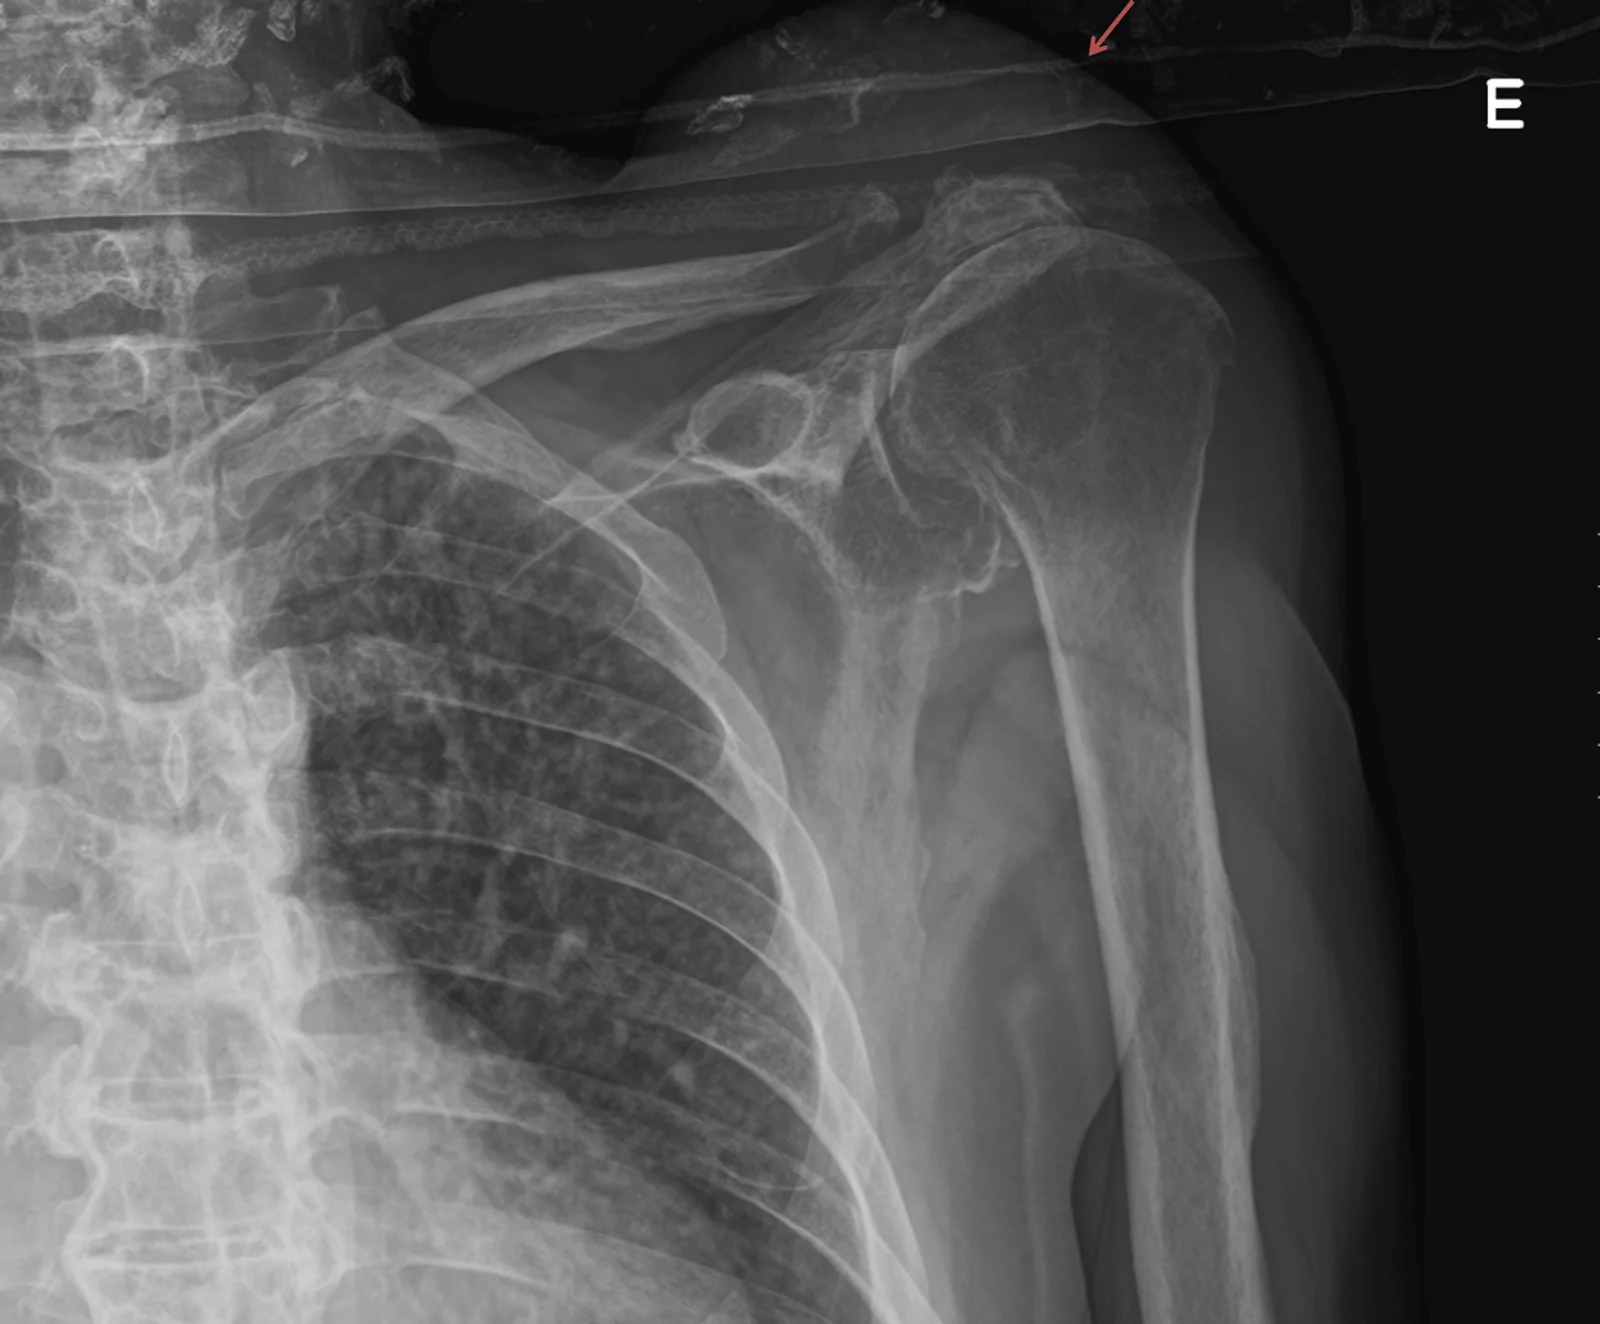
Left shoulder radiograph showing soft-tissue swelling superior to the ACJ (arrow), advanced degenerative changes of the GHJ and ACJ, superior migration of the humeral head, and narrowing of the subacromial space. ACJ, acromioclavicular joint; GHJ, glenohumeral joint
